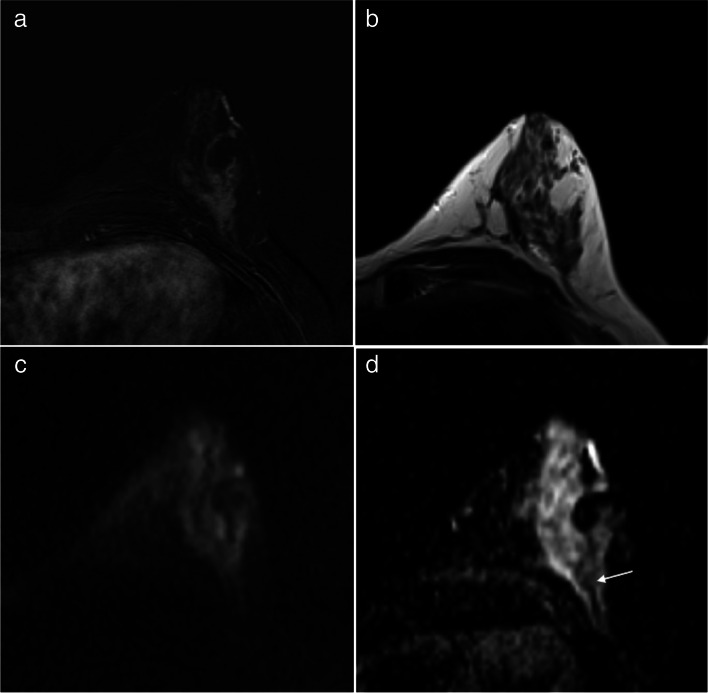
True-positive assessment of a ductal carcinoma in situ associated with invasive ductal carcinoma in a 46-year-old woman. The hypervascular left-sided lesion showed both mass and non-mass components on the first subtracted post-contrast image (a) while appearing relatively isointense to the fibroglandular background on T2-weighted imaging (b). The lesion signal was inhomogeneous on the high b-value image (c), with a markedly hyperintense area corresponding to contrast enhancement and a zone appearing as markedly hypointense compared to the surrounding tissue on the ADC map (arrow in d). Despite the inhomogeneous lesion appearance, both readers independently selected this zone for placing the ROI and obtained comparable ADC values of 0.75 e 0.72 × 10^–3^ mm^2^/s. The lesion was classified BI-RADS 5 and Likert 5 by both
